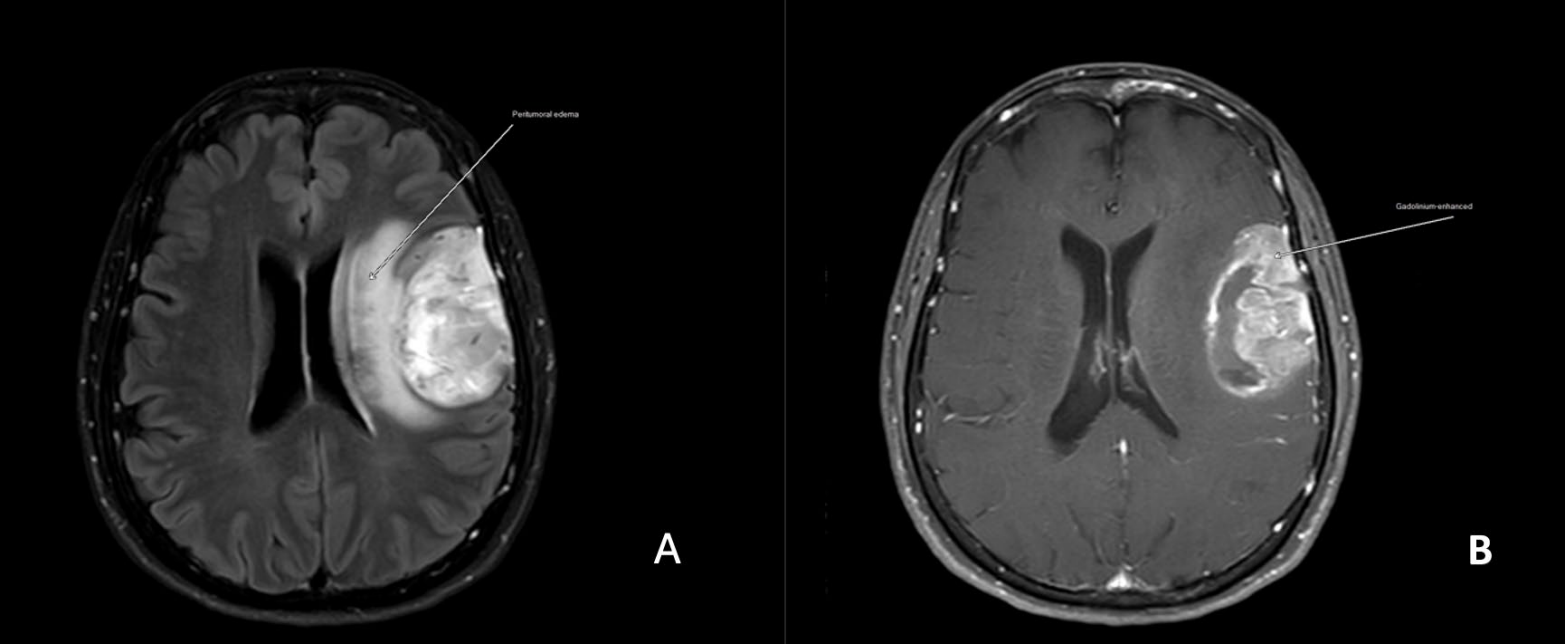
Magnetic resonance images of a patient (N11) with drug-resistant epilepsy and *GRIK2*, *GRIN3B*, *GRM3*, *GRM5*, and *GRM6* mutations (A). The axial 5-mm thick T1-weighted image shows an intra-axial mass at the left frontoparietal operculum with surrounding vasogenic edema (arrow) with heterogeneous enhancement (B).
